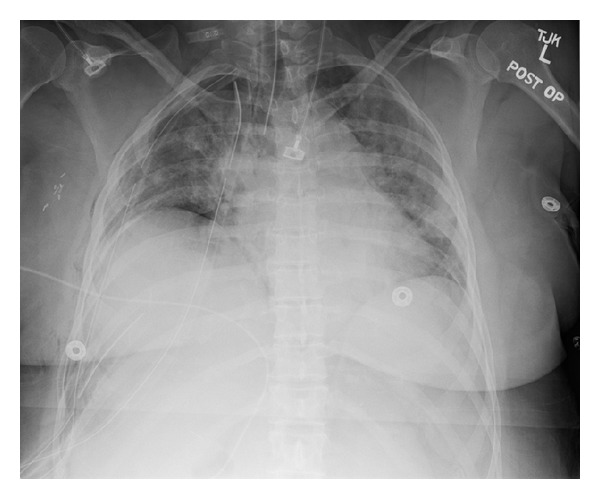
Postoperative chest X-ray. Seen are a single lumen ETT (DLT was replaced with SLT for bronchoscopy), three right chest tubes, and tiny right apical and basilar pneumothoraces. Infiltrate is observed throughout both lungs.
